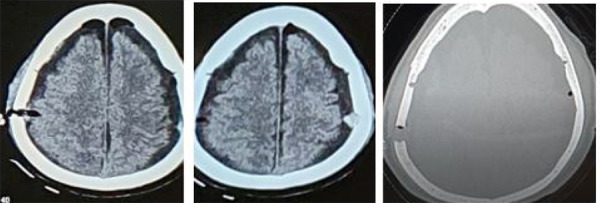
Follow up head CT after second surgery showing resolution of hematoma.
